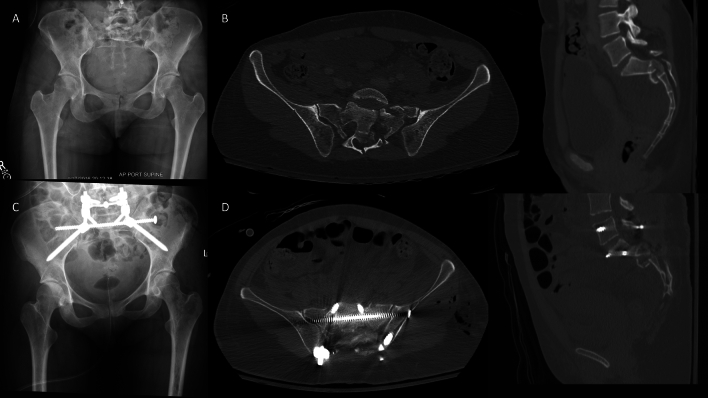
U-type sacral fracture with significant displacement treated with lumbopelvic fixation. A Injury radiographs. B On axial and sagittal CT cuts, minimal displacement is noted, and osseous fixation pathways preserved for multiple trans-iliac trans-sacral screws in the upper sacral pathway. C Postop radiographs. D Postoperative CT
